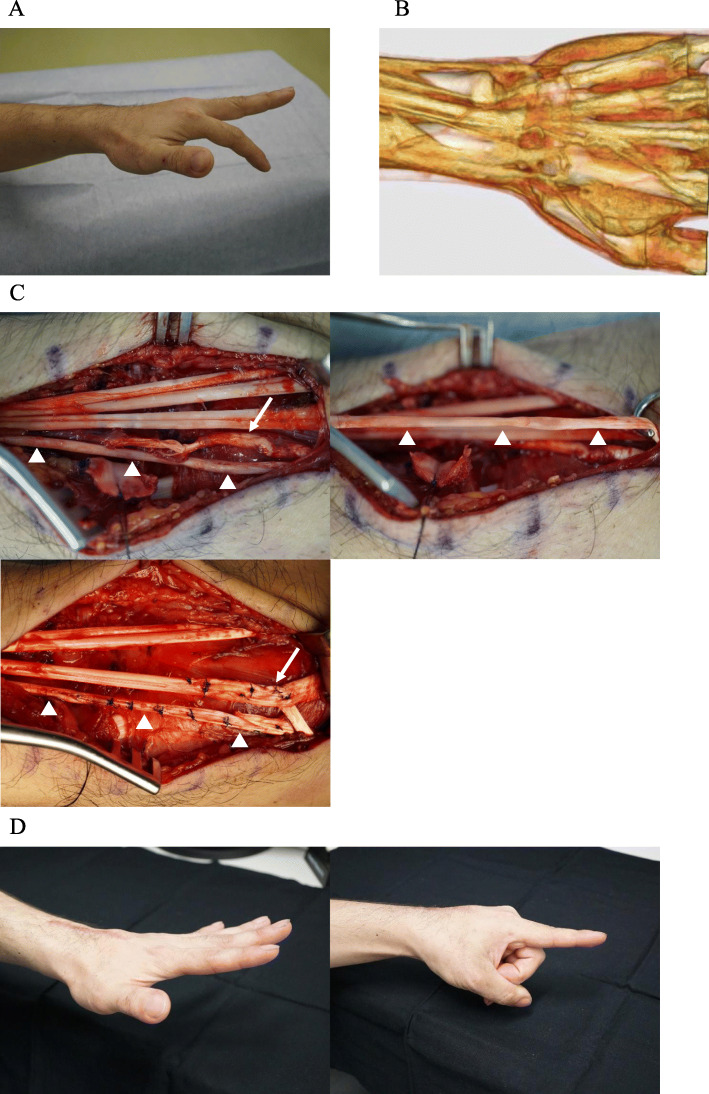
a. Preoperative extension loss of the MP joint of the left index finger. b. Three-dimensional CT imaging showing the slackness of the index EDC and EIP tendons. c. Intraoperative finding of the attenuated EIP tendon (white triangle) and partial tear of the index EDC tendon (white arrow). Shortening of the attenuated EIP tendon and tendon transfer of the index EDC to the middle EDC tendon. d. Postoperative full extension of the MP joint of the left index finger
